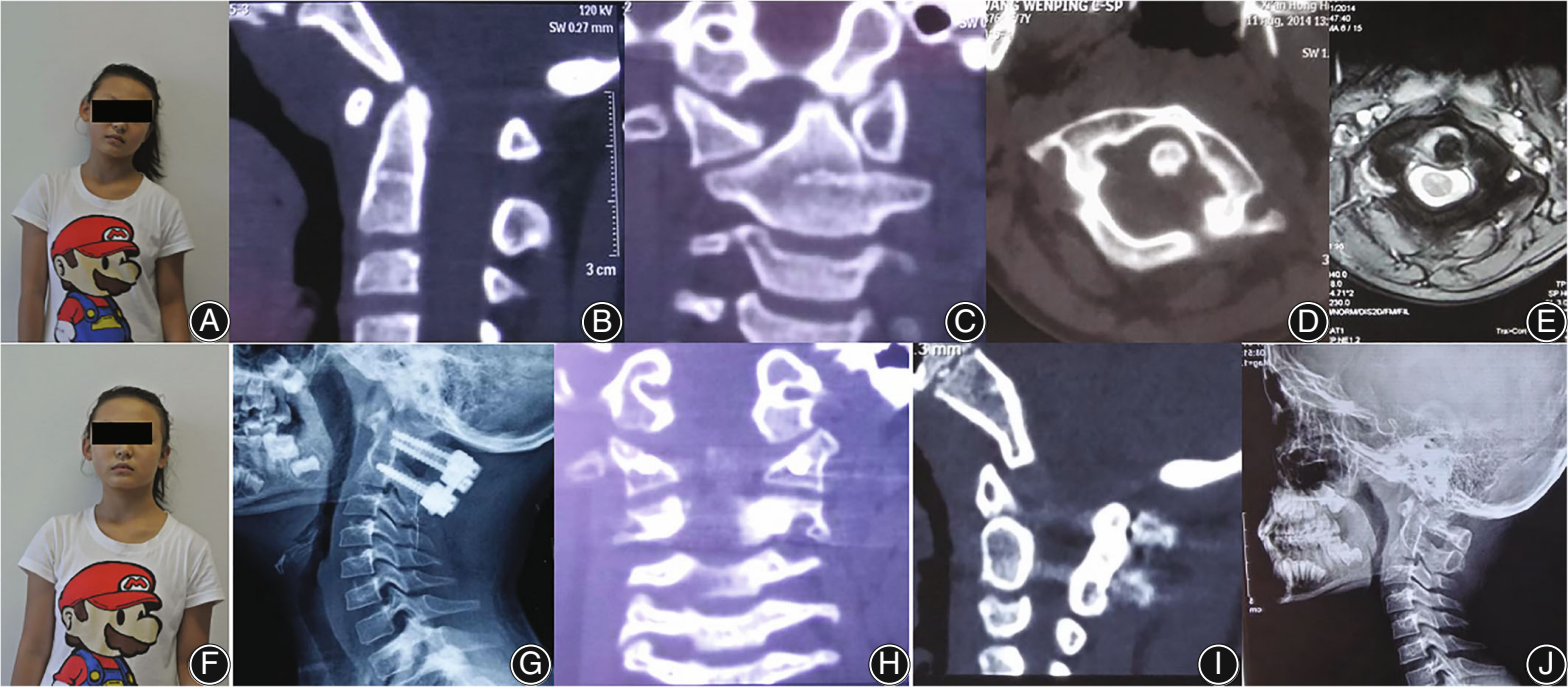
The female, 11 years old, was found to be torticollis for 5 months. (A) The patient showed left torticollis. (B–D) Preoperative CT suggested rotational fixation of atlantoaxial vertebrae, and ADI was 4.3 mm. (E) Axial MRI indicates relaxation of transverse ligament. (F) Postoperative torticollis correction. (G) Post operation 3 days, lateral X‐ray showed that ADI decreased to 2.3mm. (H) Coronal CT post operation 3 months showed that the orthopaedics were maintained well. (I) Post operation 1 year, CT showed bone graft fusion. (J) Post operation one and a half years, there was no loss after internal fixation.
